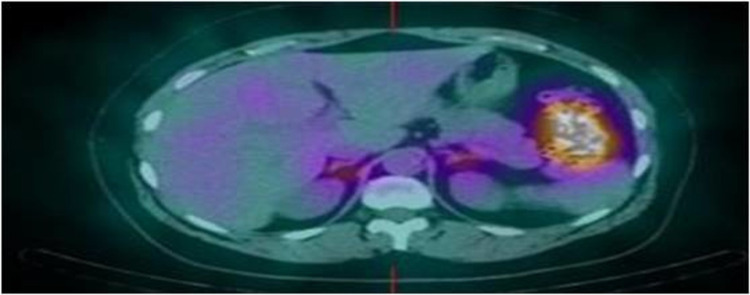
SPECT-CT: Bilateral metabolic activity increase in both adrenal glands.
